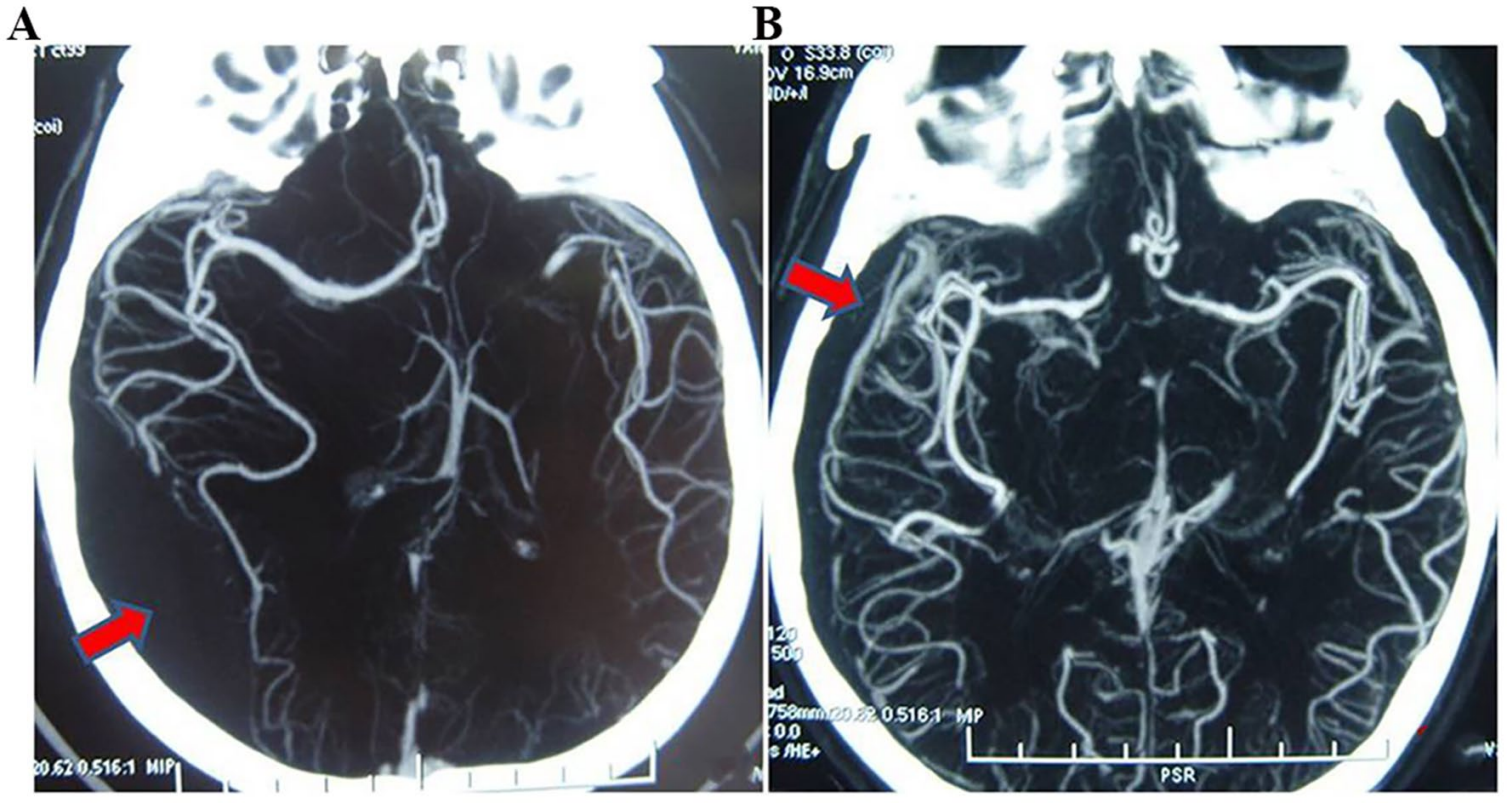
CS image of CTA. Two cases of severe TBI patients. (A) Acute epidural hematoma leading to CS; (B) acute subdural hematoma leading to CS. The red arrow indicates the hematoma.
